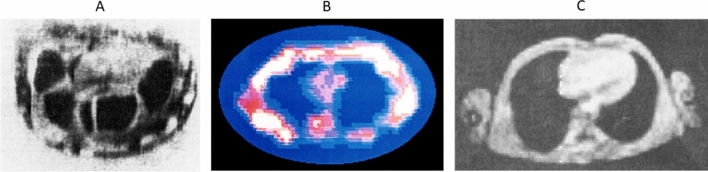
Early MR images: A first MRI image of a human wrist (from Fig. 1, ref. [8]) acquired with the sensitive line method on a 0.7 T magnet in 9 min. B Image of a human thorax acquired with the FONAR method (from ref. [9]). The image was acquired in a superconductive magnet with 0.0508 T by moving the subject to 64 different positions inside of a huge magnet, total acquisition time was 4 h 45 min. C Spin-warp image from a human thorax (from Fig. 2 ref. [17]) acquired at 0.04 T in 64 s
